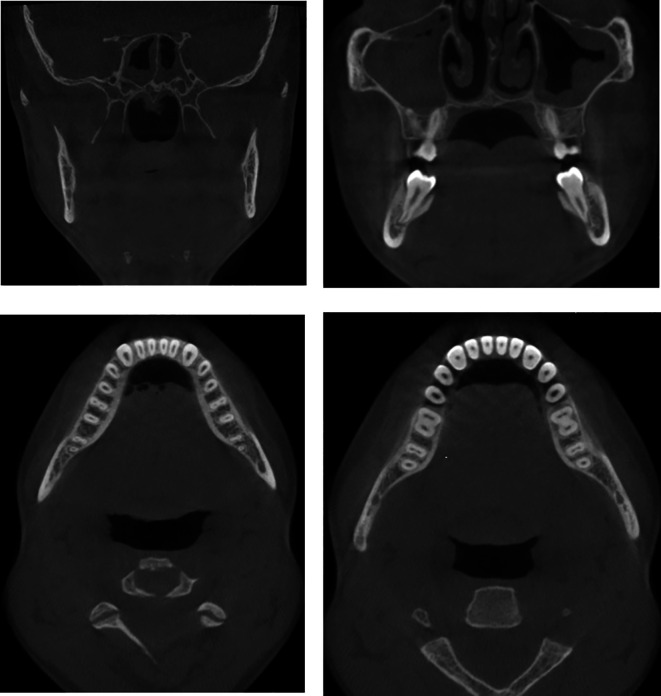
Postoperative CBCT showing erupted premolars, extracted molar site, and normal bone contour.
